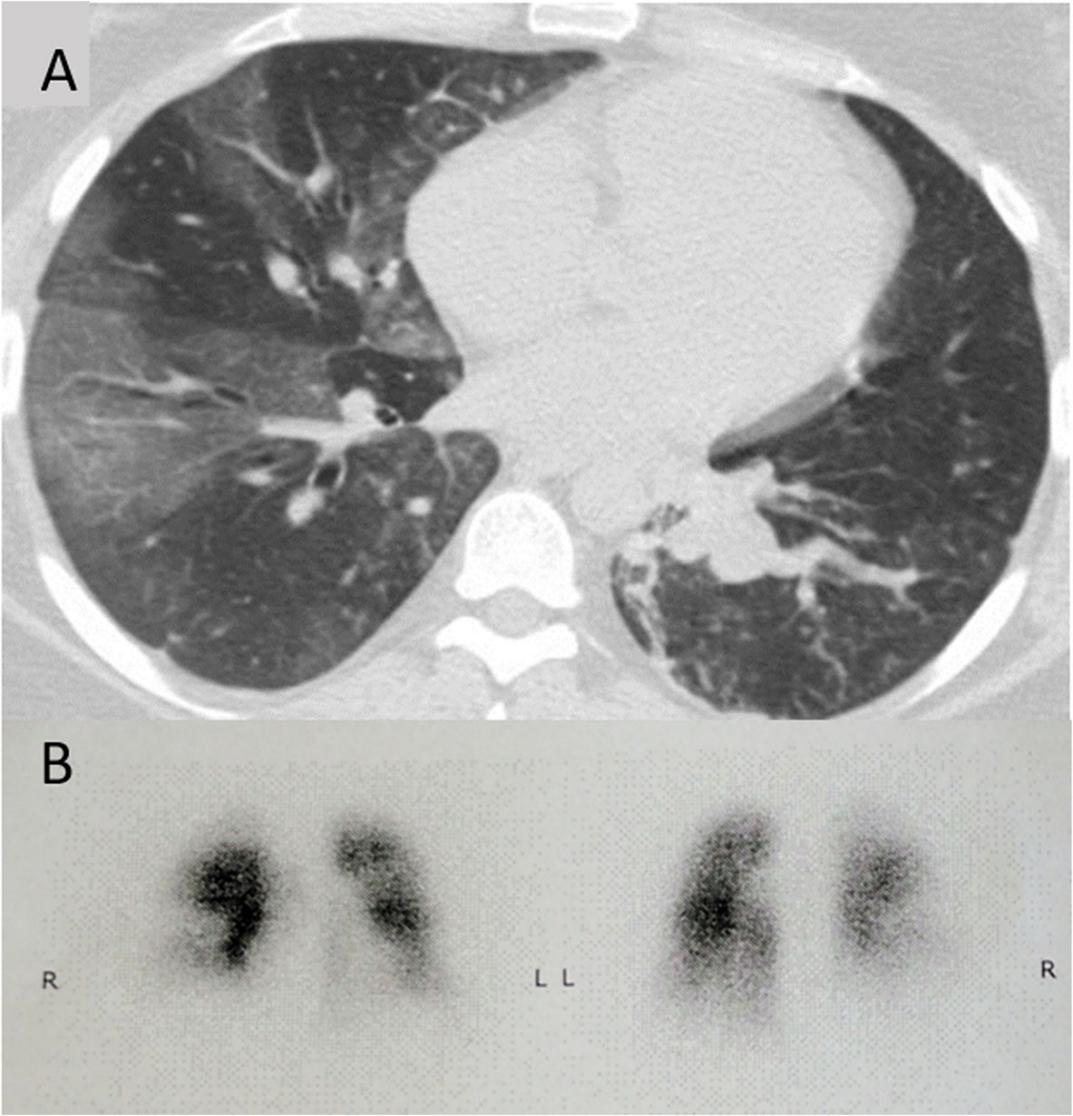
a Follow up chest CT scan showing obstruction of right lower lobe bronchi by blood clots as well as enlarged pulmonary vessels and mosaic pattern of lung parenchyma. b Anterior-posterior and posterior-anterior view of ventilation/perfusion scan showing bilateral perfusion deficits
